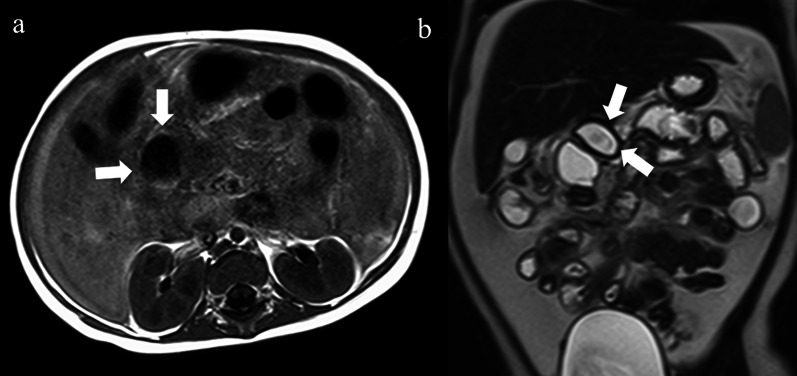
MRI findings. The cyst (white arrows) showed low intensity on T1-weighted magnetic resonance imaging (MRI) (a) and high intensity on T2-weighted MRI (b)
